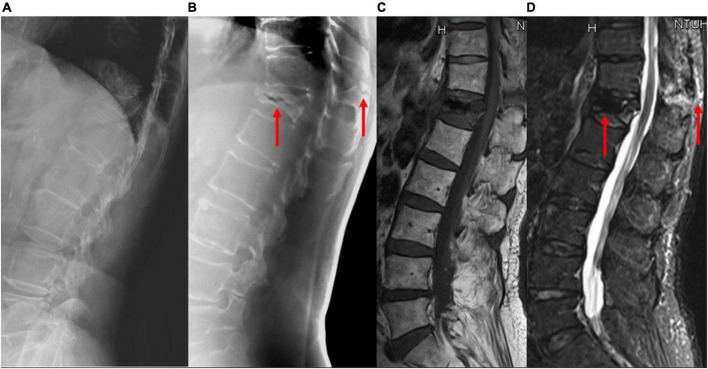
A patient with acute VCF at the T-L junction. The bony details of the fracture and the adjacent vertebral levels are ruined by the abrupt density transition from the lungs to the abdomen and the decreased bone mineral density on (A) lateral radiographs; (B) tomosynthesis; (C) T1-weighted MRI, and (D) short tau inversion recovery MRI images clearly show the fracture involving the anterior and posterior vertebral edges and the spinous process (arrows). VCF, vertebral compression fracture; T-L, thoracolumbar; MRI, magnetic resonance imaging.
